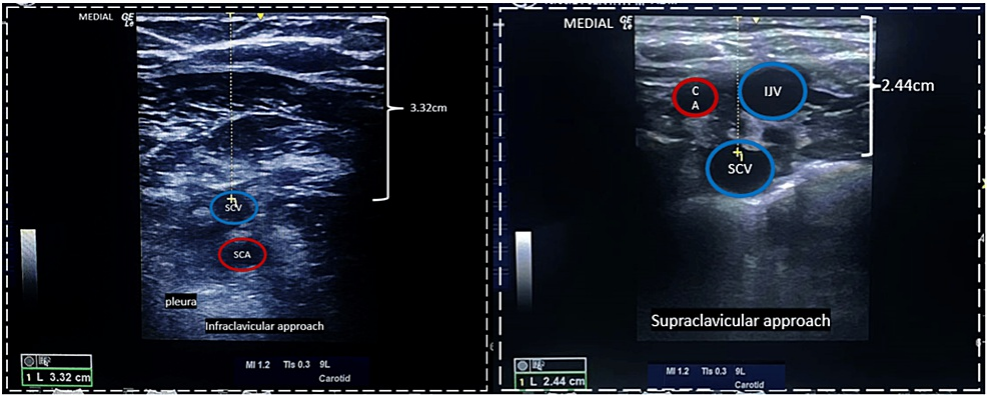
Anatomical marking from the probe to the vein in real-time ultrasound showing the distance difference between the infraclavicular and supraclavicular approach of subclavian vein access The anatomical landmark of the subclavian vein; the distance between the probe and the subclavian vein in the infraclavicular approach is 3.32 cm. The distance between the probe and the subclavian vein in the supraclavicular approach is 2.44 cm. Red color marking (SCA: subclavian artery and CA: carotid artery); blue color marking (IJV: internal jugular vein and SCV: subclavian vein) (original image)
